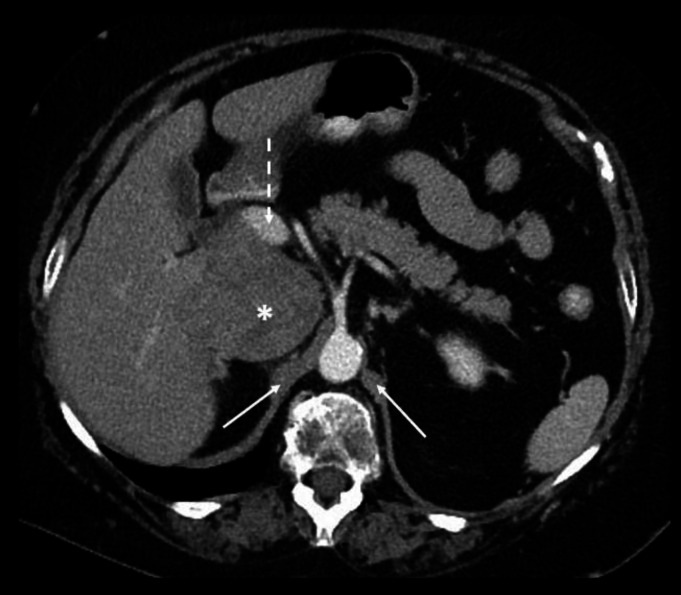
Contrast enhanced axial CT abdomen of a 80-year-old female demonstrates a large, expansile, soft-tissue density mass (asterisk) posterior to the portal vein (dashed white arrows). Review of other images show the mass to be separate from the liver but distinction between this mass and the IVC was not possible. The mass was diagnosed to be leiomyosarcoma arising from the IVC following surgery. It shall be remembered that the origin or epicentre of a mass may not be confidently determined on a single image and necessitates review of series of images in different orthogonal planes. Right and left crus of diaphragm are indicated by solid white arrows
